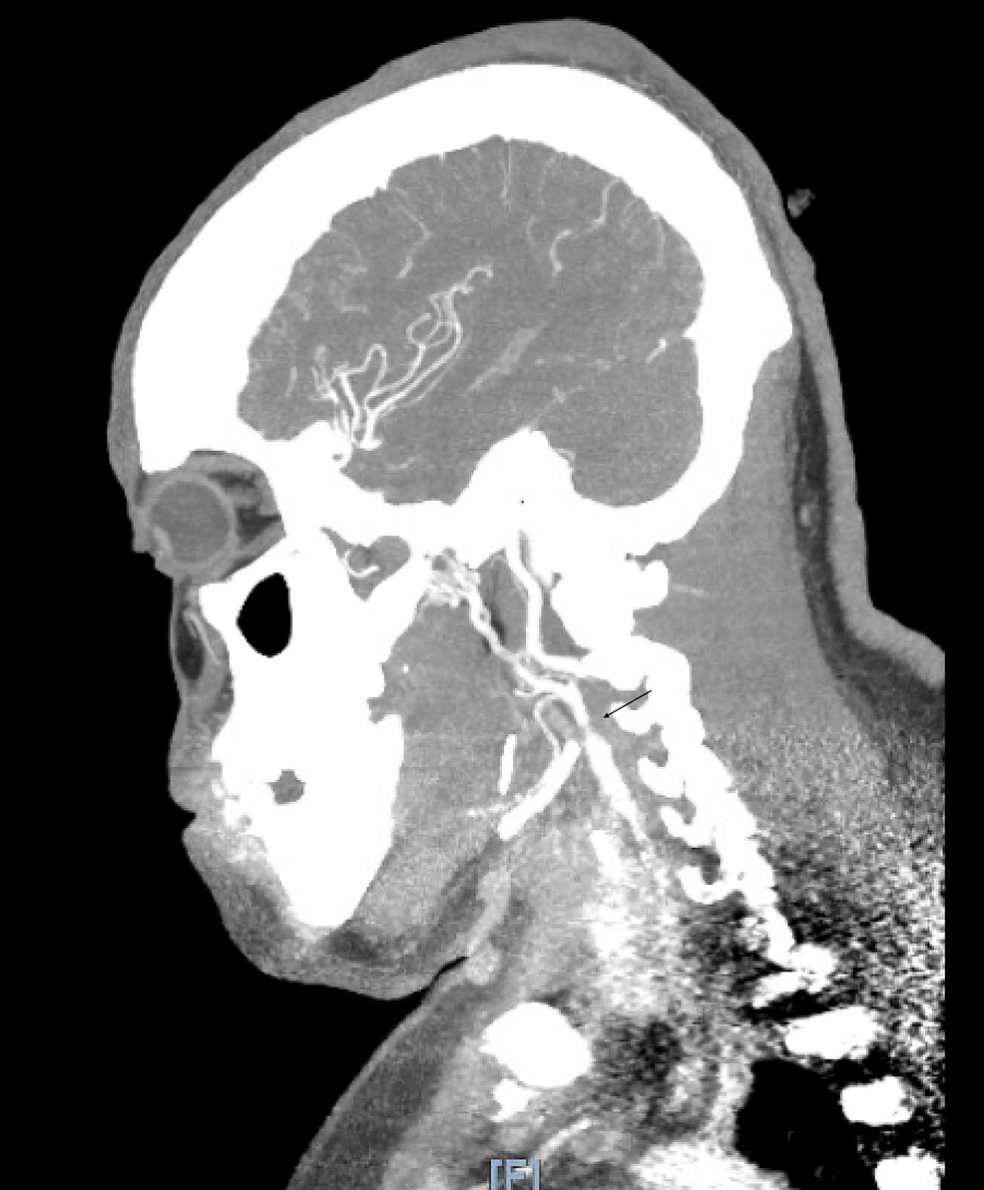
A sagittal image of CTA head and neck, arrow showing stenosis in proximal ICA CTA: CT angiography; ICA: Internal carotid artery.
